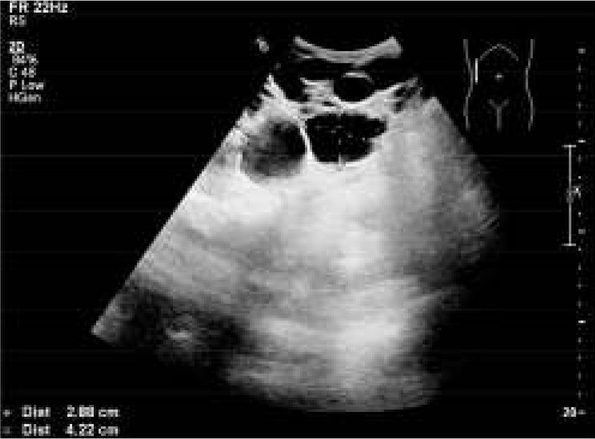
Ultrasonography shows right kidney with many cysts up to 42 mm in diameter.
